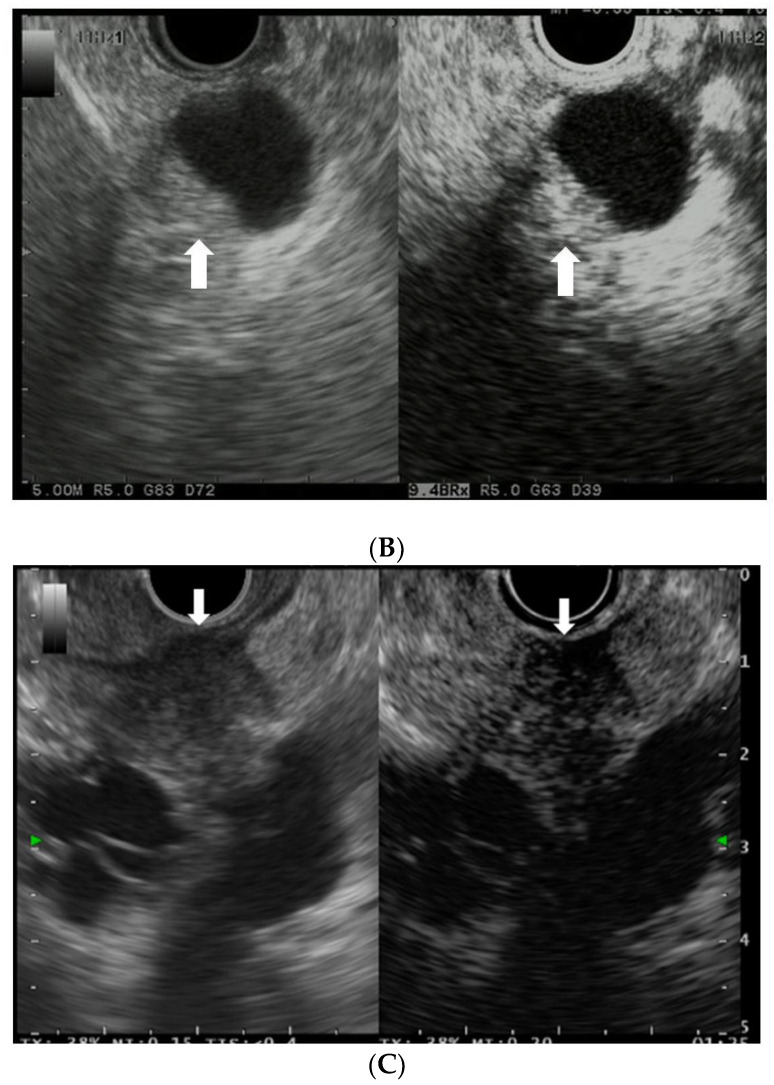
(A) Representative case of a mucus clot without vascularity. Conventional endoscopic ultrasonography (EUS; left) reveals an isoechoic mural lesion (arrow) in a cyst. Contrast-enhanced harmonic EUS (right) reveals a mural lesion (arrow) without vascularity. (B) Representative case of a mural nodule with vascularity. Conventional endoscopic ultrasonography (EUS; left) reveals an isoechoic mural lesion (arrow) in a cyst. Contrast-enhanced EUS (right) reveals a mural lesion (arrow) with vascularity. (C) Representative case of an invasive IPMC. Conventional endoscopic ultrasonography (EUS; left) reveals a hypoechoic lesion (arrow). Contrast-enhanced EUS (right) reveals a hypoenhancing lesion (arrow) due to early wash-out.
